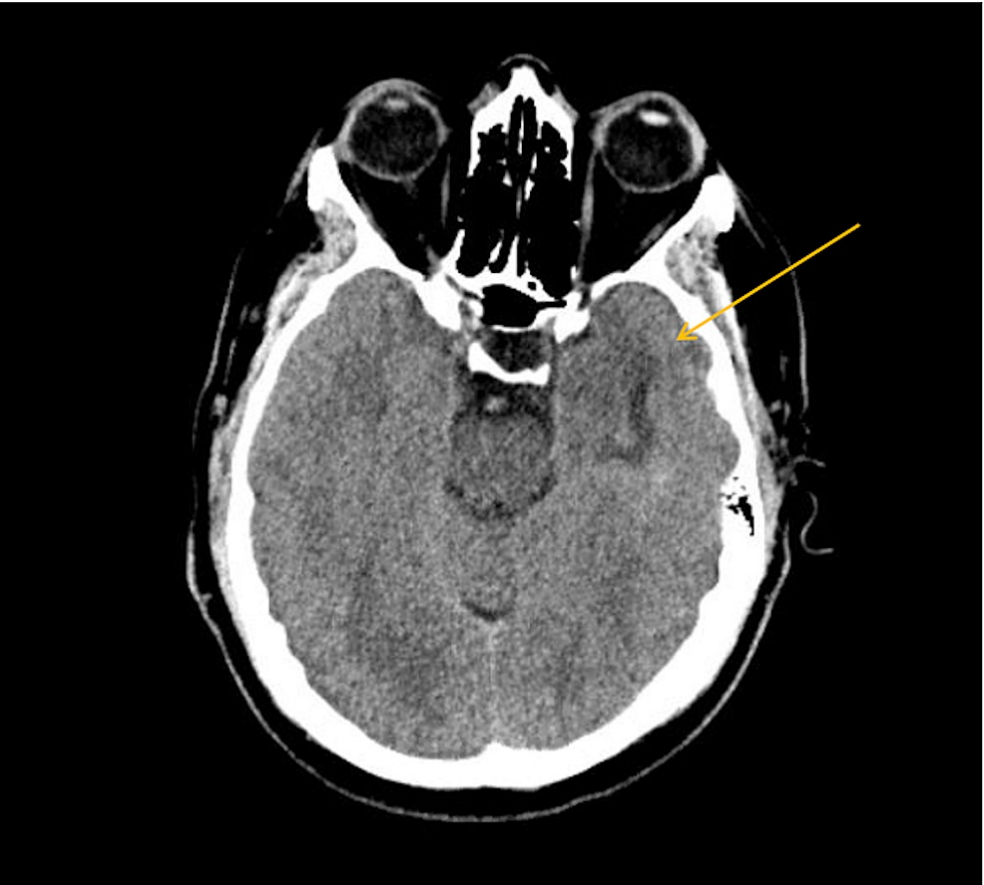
CT of the head showing chronic, small area of encephalomalacia in the left temporal lobe (yellow arrow).
